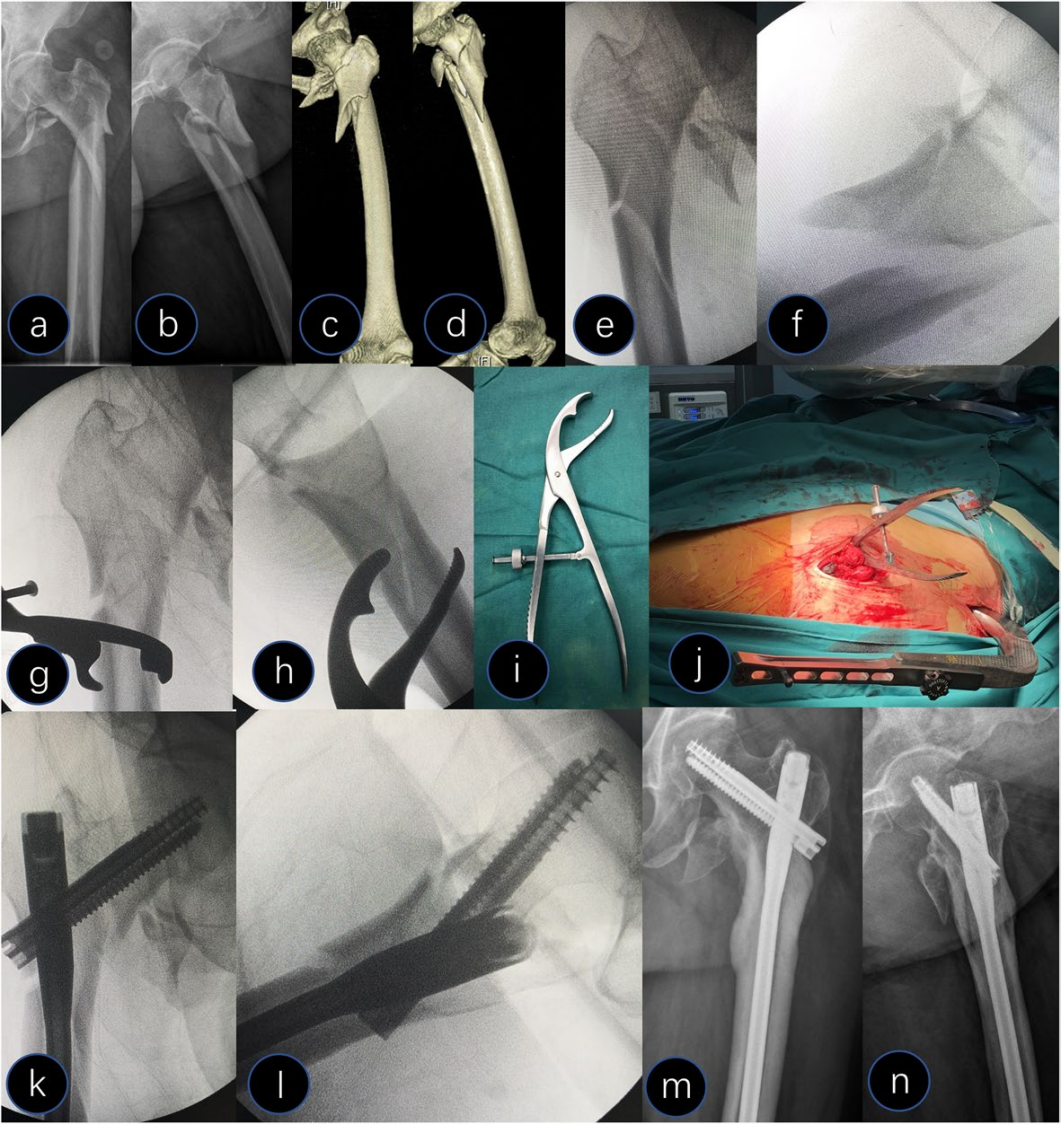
A 65-year-old female presented with a Type V fracture. a-d Preoperative X-ray and computed tomography 3D reconstruction showed an anterior-posterior displacement of the subtrochanteric region. e and f Closed reduction with traction under fluoroscopy. g and h A two-jaw clamp was used to reduce the subtrochanteric fracture. i Picture of the two-jaw clamp. j Surgical pictures of the clamp placement. k and l Intraoperative X-ray after reduction and fixation. m and n Postoperative X-ray at 13 months
